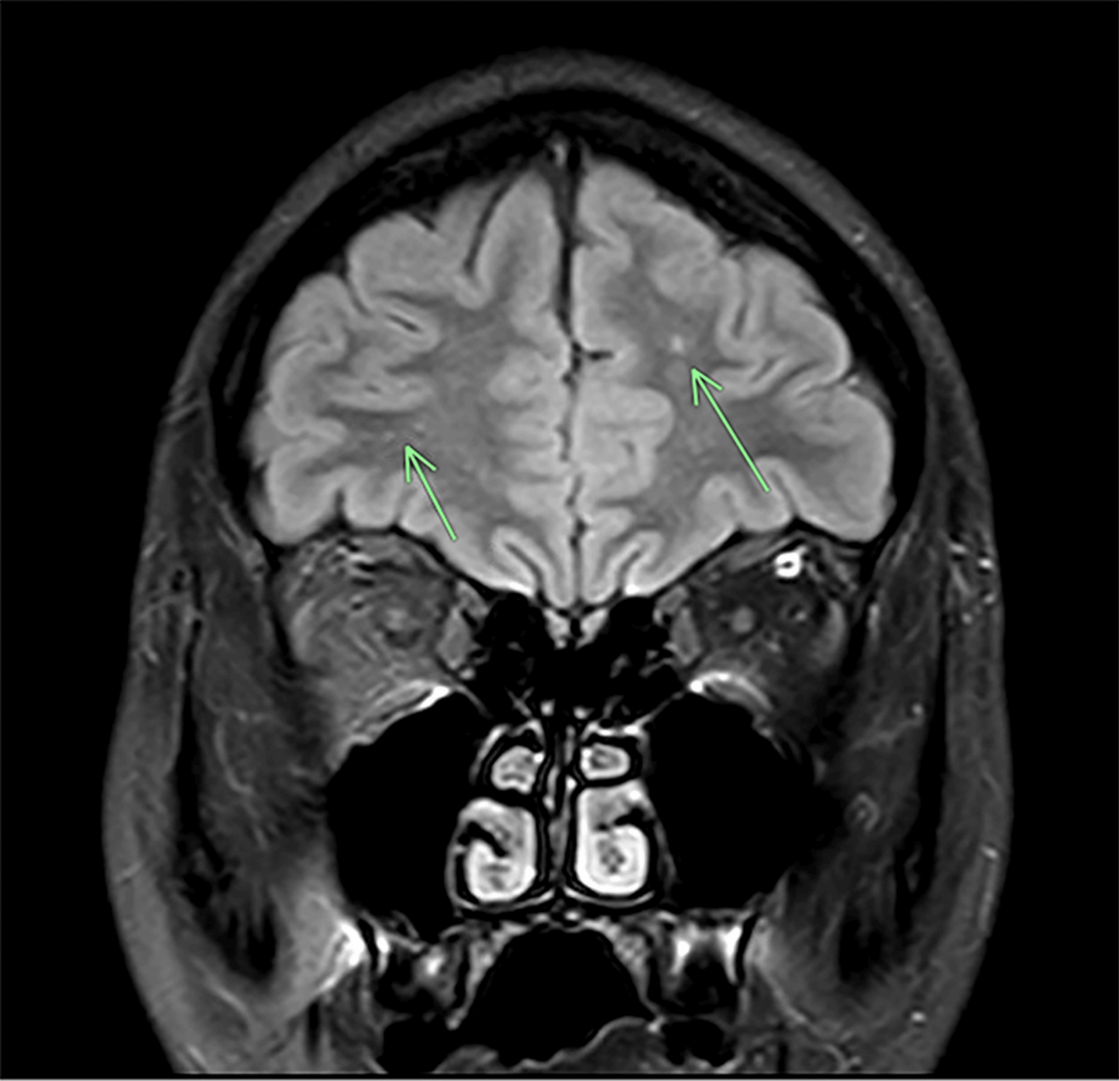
MRI brain, coronal T2-FLAIR. The arrows indicate nonspecific punctate T2-FLAIR foci in the bilateral frontal lobes. T2-FLAIR: T2-weighted fluid-attenuated inversion recovery.
